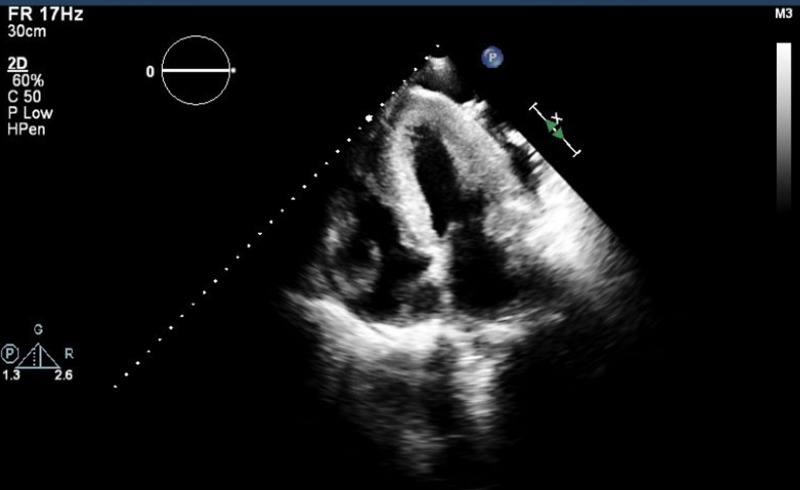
Transthoracic echocardiography four chamber view Severe concentric biventricular hypertrophy
